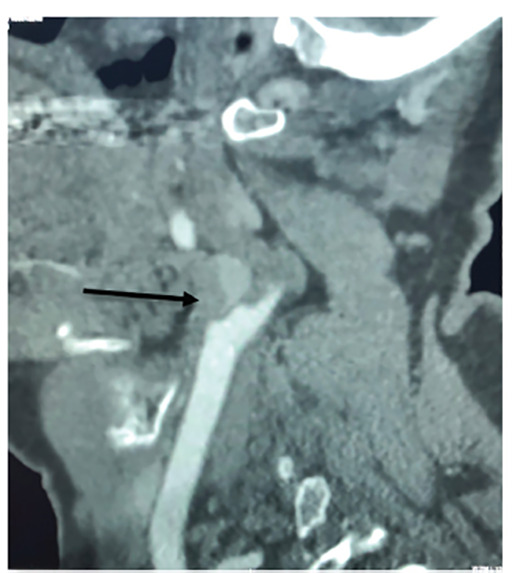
Computed tomography demonstrating complete occlusion of the left internal carotid artery depicting “flame occlusion” (arrow).
